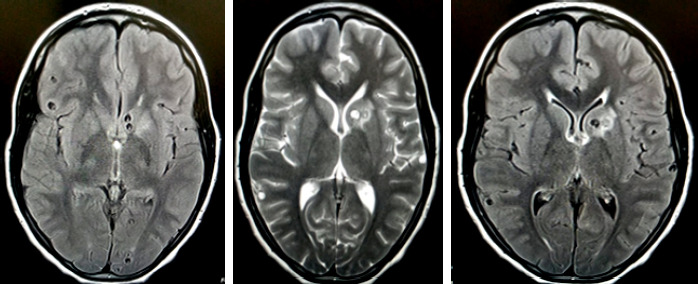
IRM cérébrale mettant en évidence de multiples images kystiques arrondies en hyposignal pondéré T1, hypersignal pondéré T2 et en hyposignal FLAIR de localisation éparse en cortico-sous corticale
